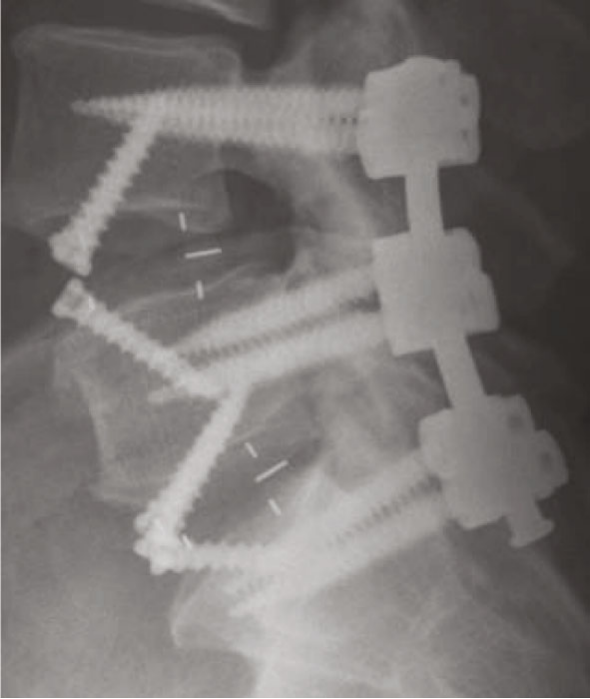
Postoperative lateral X-ray.
